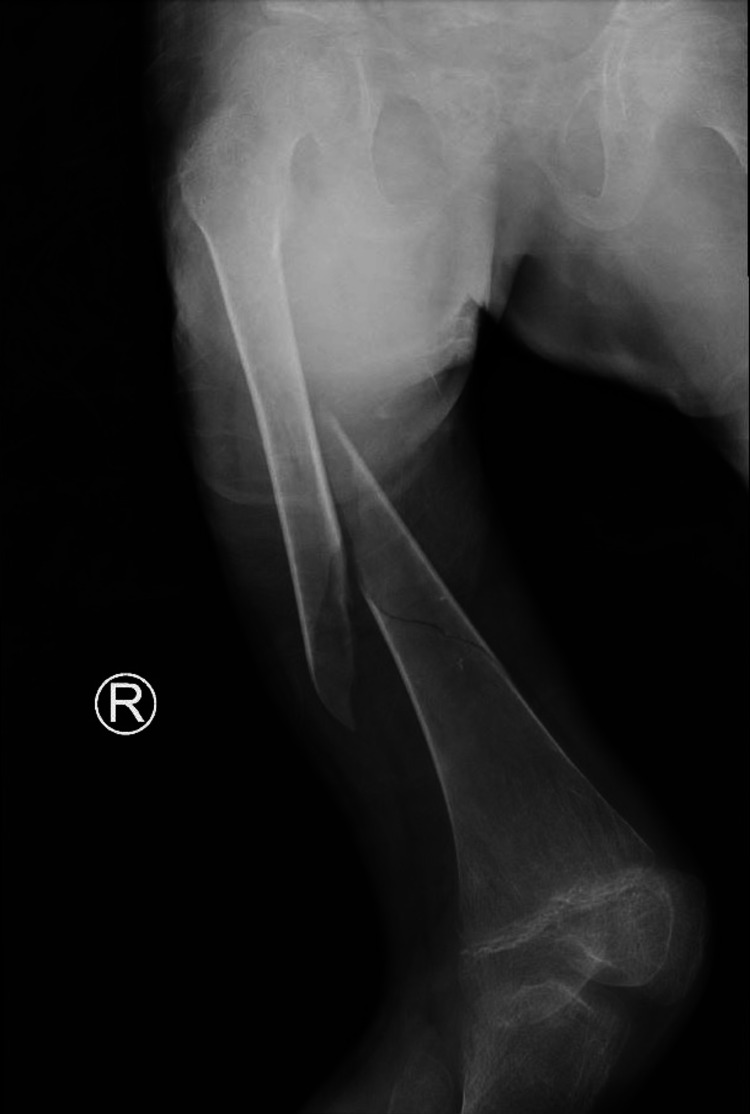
X-ray image taken on the day of the admission showing the hip, femur, and knee of a skeletally immature patient. A right femur spiral fracture in the mid-diaphyseal region can be seen along with a deformity that cannot be reduced due to epidermolysis bullosa and the fear of sloughing off the skin due to the nature of the disease. Narcotics and analgesia were administered and the patient was left in the best-preferred position for maximum comfort.
